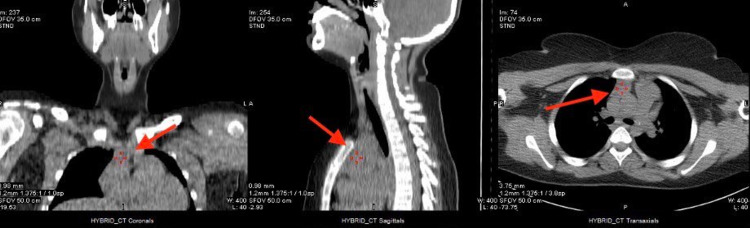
CT scan showing a hyperdense lesion in the anterior mediastinum imbedded in the thymus gland.
